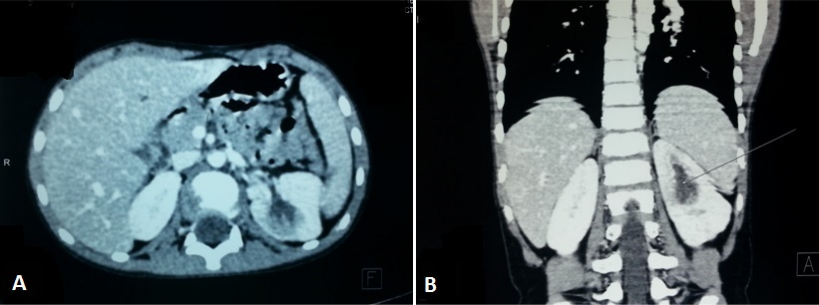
Computed tomographic images showing a heterogeneous left renal mass measuring 40/36/30 mm: A) scannographic axial section; B) frontal reconstruction
